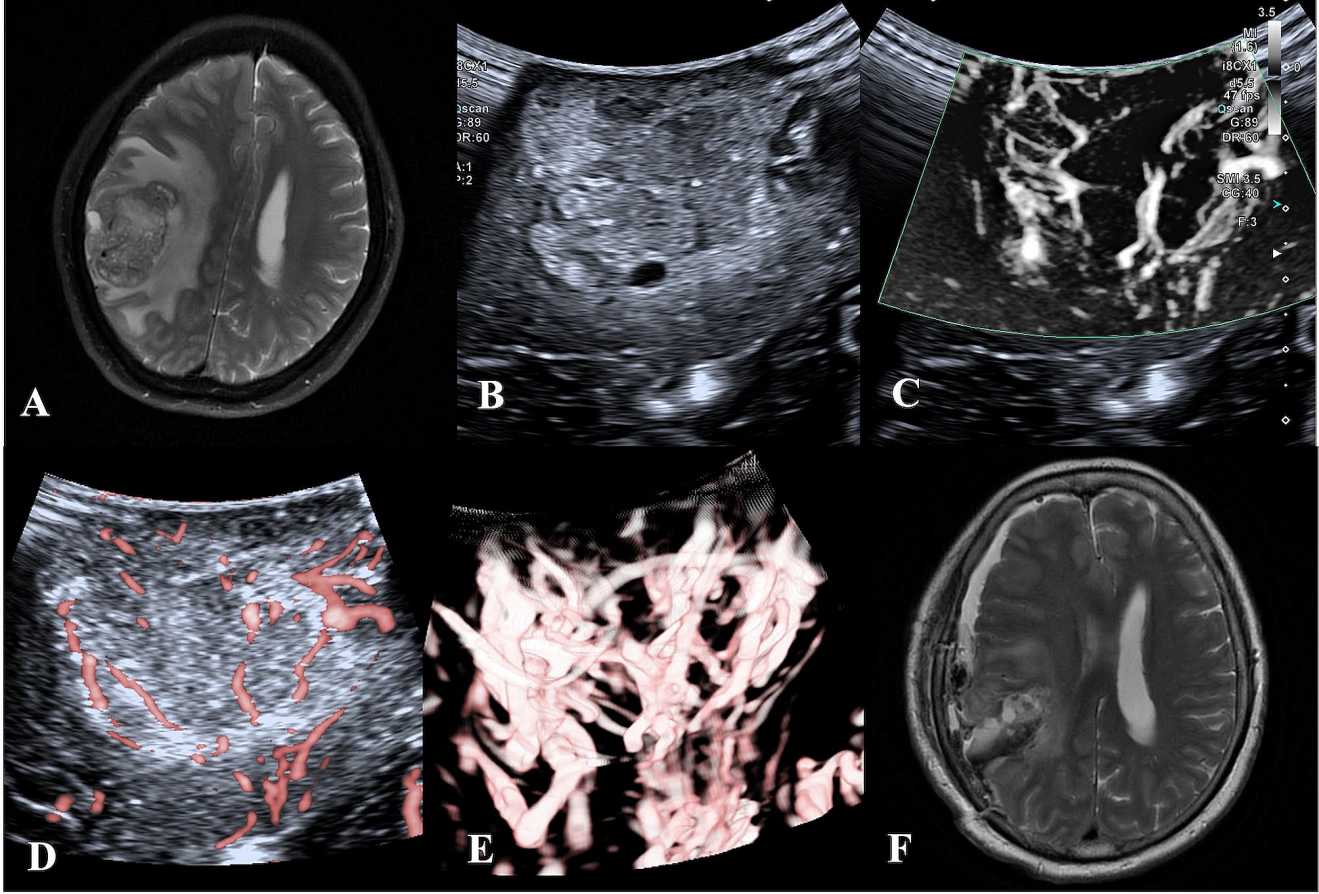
MRI, B-mode, and SMI of HGG in a 65-year-old woman. (A) Preoperative MRI T2W showed that the parietal lobe lesion showed high signal, irregular, blurred boundary, and large edema surrounding. (B) B-mode ultrasound, mixed echoes can be seen in the parietal lobe, irregular shape, unclear boundary, and peripheral edema. B-D. SMI mode, B is mSMI mode, C is cSMI mode, and D is Smart 3D-SMI mode. The vascular architecture in tumor parenchyma is extremely dilated, blood vessels are distorted and disordered, and the tumor boundary display is more transparent than that of B-mode ultrasound. F. Postoperative MRI T2W showed fluid and air accumulation in the operative area after resectioning parietal cystic lesions
